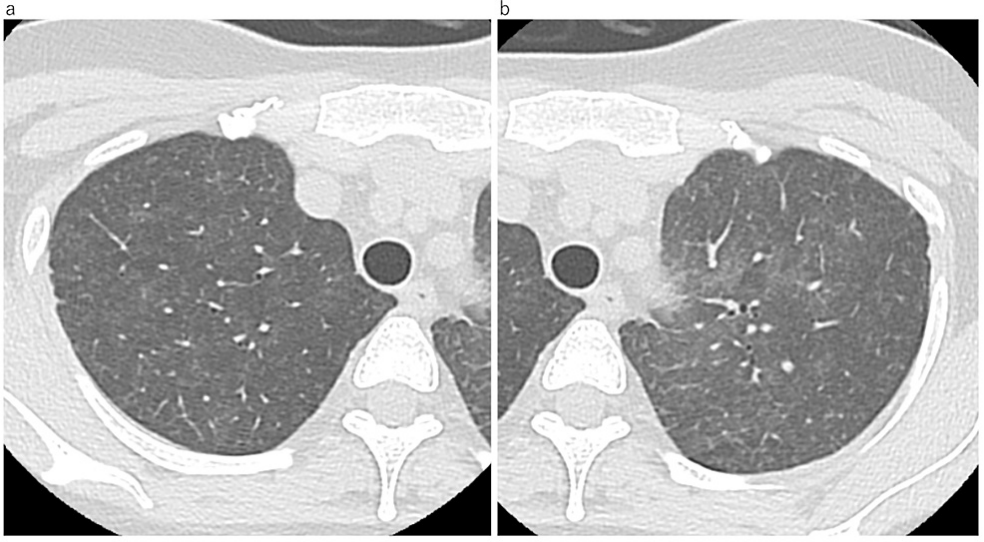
High-resolution computed tomography (HRCT) of Case 2 on admission a,b: HRCT showing slight bilateral diffuse ground-glass opacity.
